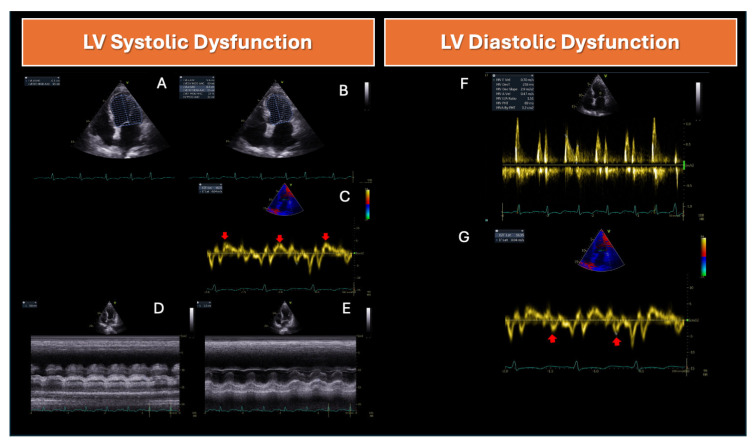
Echocardiographic markers of left ventricular systolic and diastolic function. (A,B): Left ventricular ejection fraction (EF) estimation through the Simpson’s method in a patients with SCM. LVEF was 27%. (C): Tissue Doppler imaging evaluating the systolic velocity of the lateral mitral annulus (S’) which was very low in the present patient (0.04 m/s) (red arrows). (D,E): Mitral Annular Plane Systolic Excursion (MAPSE) at the septal and lateral mitral annulus which was 0.8 and 1.3 mm, respectively, indicating severe LV systolic dysfunction. (F,G): transmitral flow and tissue Doppler imaging at the mitral annulus to evaluate diastolic LV function. E/e’ was 16.35, severely increased, indicating diastolic dysfunction in a patient with urinary sepsis and previously normal cardiac function. E’ was 0.04 cm/s also indicating severe diastolic dysfunction.
